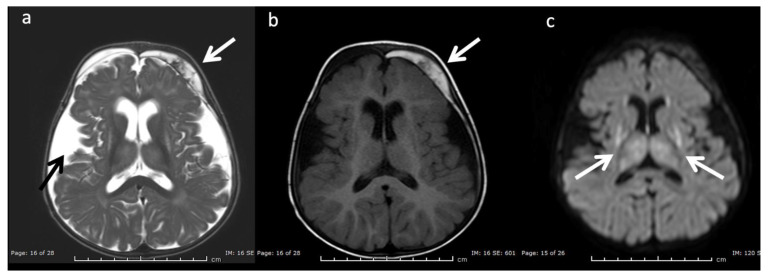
Eleven-month-old female with Glutaric aciduria Type I with acute decompensation after illness. (a) Axial T2WI, (b) T1WI, and (c) DWI at the level of the basal ganglia demonstrate diffuse cerebral volume loss, underopercularization (black arrow, a), bifrontal chronic subdural hemorrhages with superimposed subacute left frontal subdural blood products (white arrows, a and b), and reduced diffusion in the lentiform nuclei and thalami (white arrows, c).
